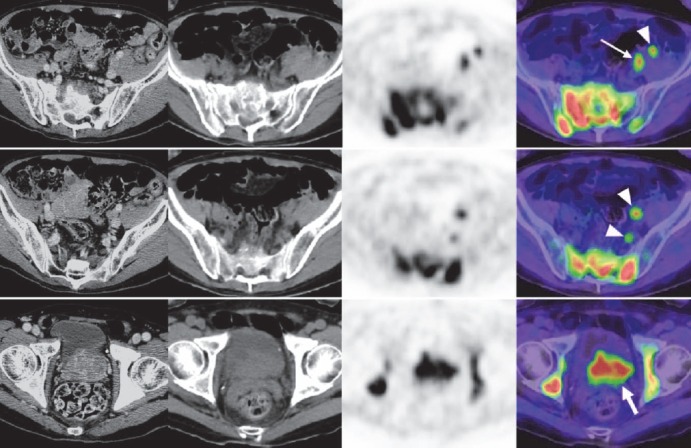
Axial images of the pelvis in a patient with lymph node metastasis and multiple bone metastases (patient no. 2 in Table 1). From left to right, contrast-enhanced thin-slice CT images, plain CT images of anti-^18^F-FACBC PET/CT, PET images, and PET/CT fusion images are presented. Images at a given body level are presented in a row. Contrast-enhanced CT and PET/CT were performed on different occasions. The primary site (thick arrow), a significantly enlarged lymph node in the left external iliac region (thin arrow), unenlarged lymph nodes in the left external and internal iliac regions (arrowheads), and multiple bone lesions showed increased uptake
